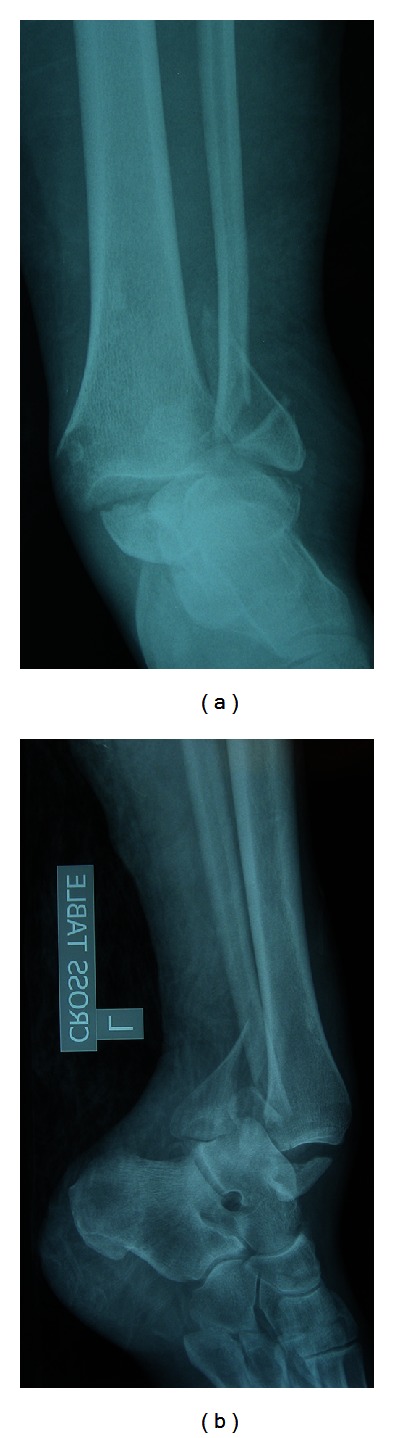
Initial anteroposterior and lateral radiographs showing an ankle fracture dislocation with lateral and posterior displacement of the talus.
